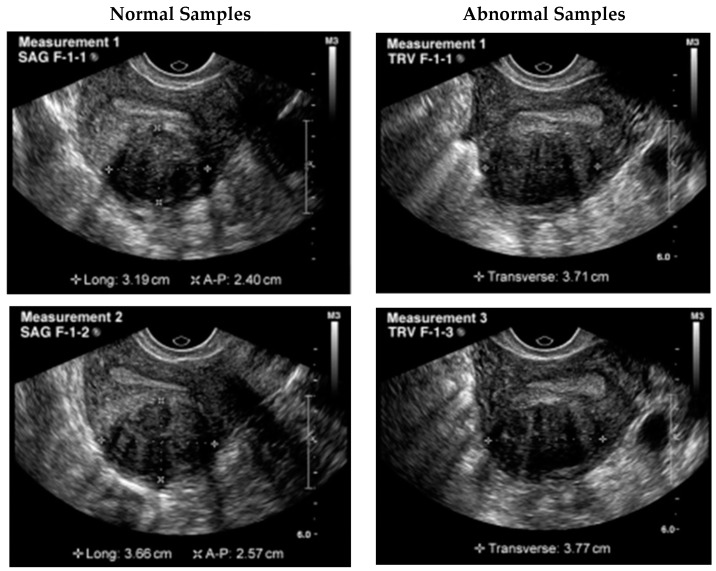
Sample ultrasound images from the dataset.
